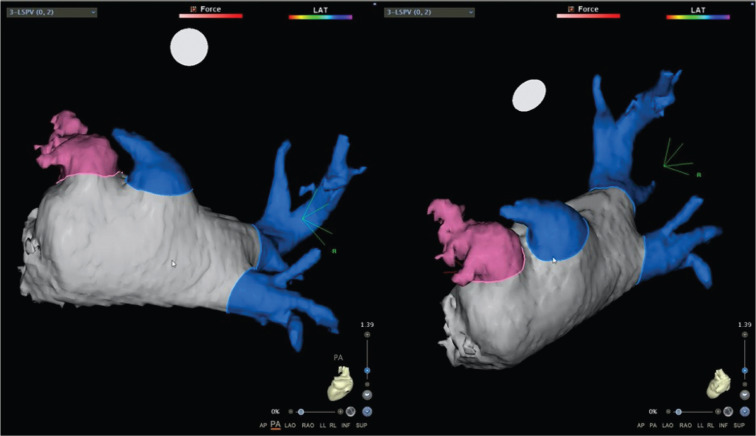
Cardiac CT in the posteroanterior and left lateral projections. The left atrial appendage is colored pink, and the veins are colored blue.
